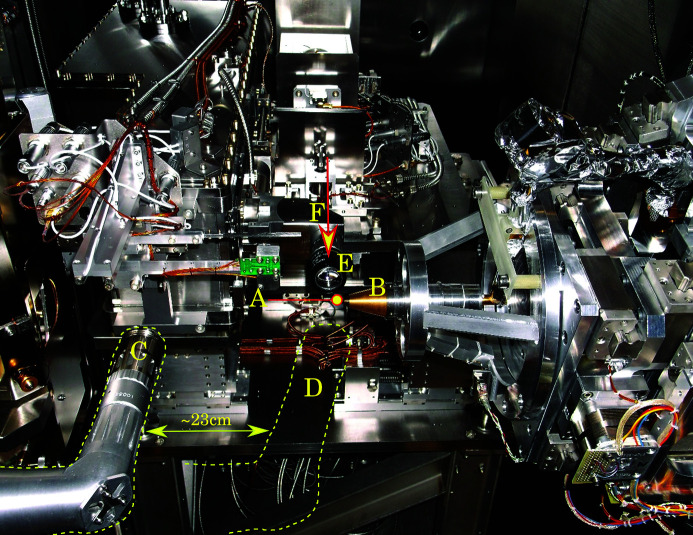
A: Sample position; B: goniometer; C: tomography camera, retracted position; D: tomography camera, in-beam position; E: on-axis viewing system; F: X-ray beam direction.
